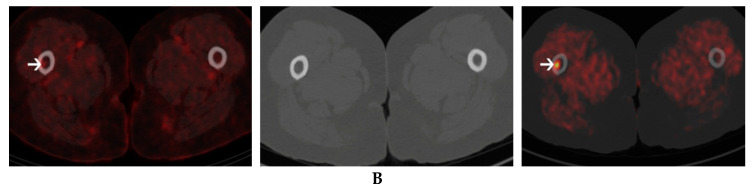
A 56-year-old female with IgG kappa MM. ^18^FDG PET/CT (left panel) and ^18^F-Choline PET/CT (right panel) were performed with a 3-day-interval. An axial PET/CT image of the pelvis shows a focal uptake of ^18^F-Choline within the right ischium without a corresponding uptake on ^18^FDG PET/CT. The corresponding axial CT image (central panel) shows no clear-cut structural changes (A). A focal lesion of the right femur exhibits a high uptake of ^18^F-Choline. This lesion exhibits only a faint uptake on ^18^FDG PET/CT (left panel) and is not visible on CT (central panel) (B).
